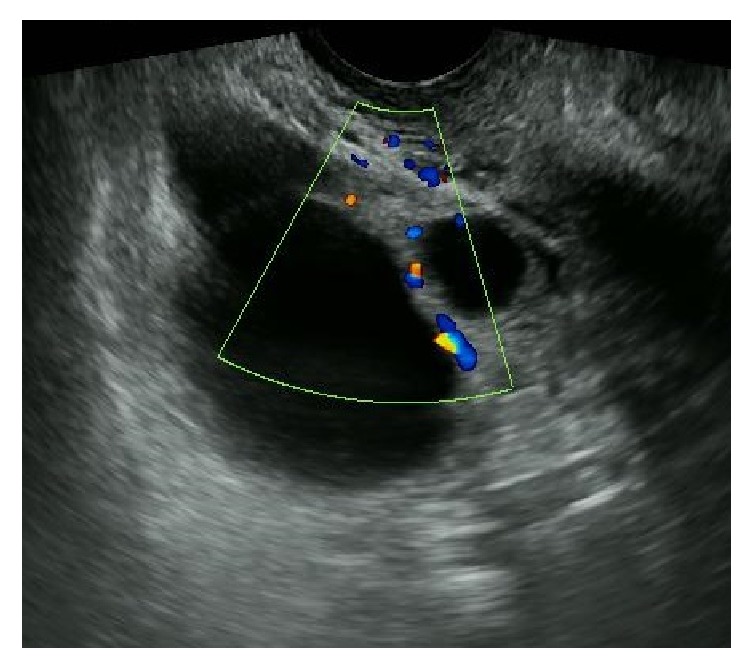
The cystic tumor of the right ovary had neovascularity demonstrated by color Doppler ultrasonography.
